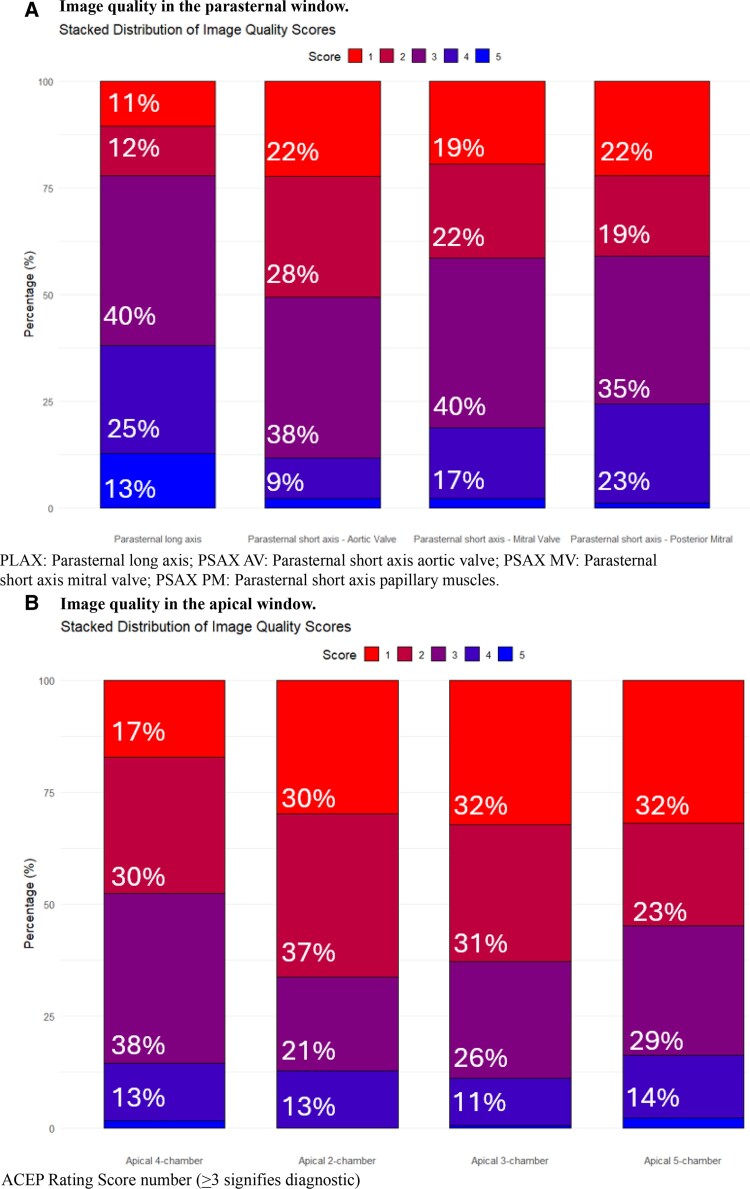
(*A*) Image quality in the parasternal window. PLAX, parasternal long-axis; PSAX AV, parasternal short-axis aortic valve; PSAX MV, parasternal short-axis mitral valve; PSAX PM, parasternal short-axis papillary muscles. (*B*) Image quality in the apical window. ACEP Rating Score number (≥3 signifies diagnostic).
